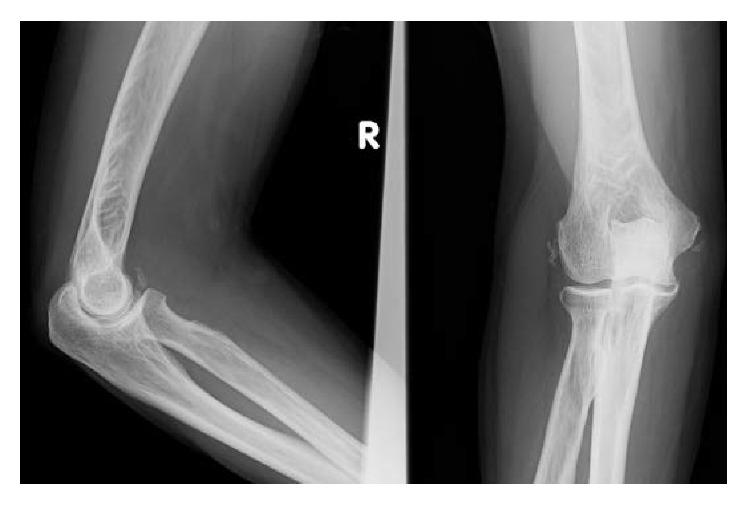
Eight weeks after the trauma, radiographs revealed mild calcification around the anterior articular capsule, medial collateral ligament, and lateral collateral ligament.
